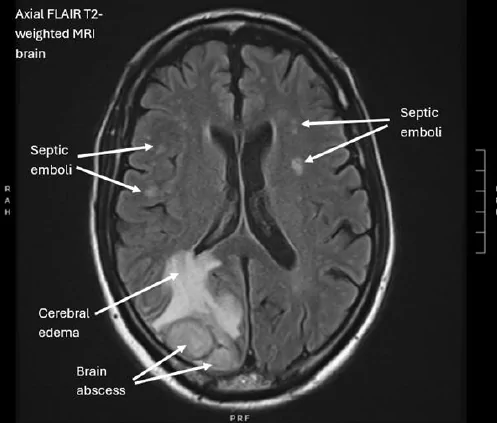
Axial fluid attenuated inversion recovery brain magnetic resonance image showing increased T2-weighted signal in the posterior right frontal lobe and right parietal-occipital region with mild to moderate localized edema and mild mass effect but no midline shift, suspicious for multifocal brain abscess (white arrows).
